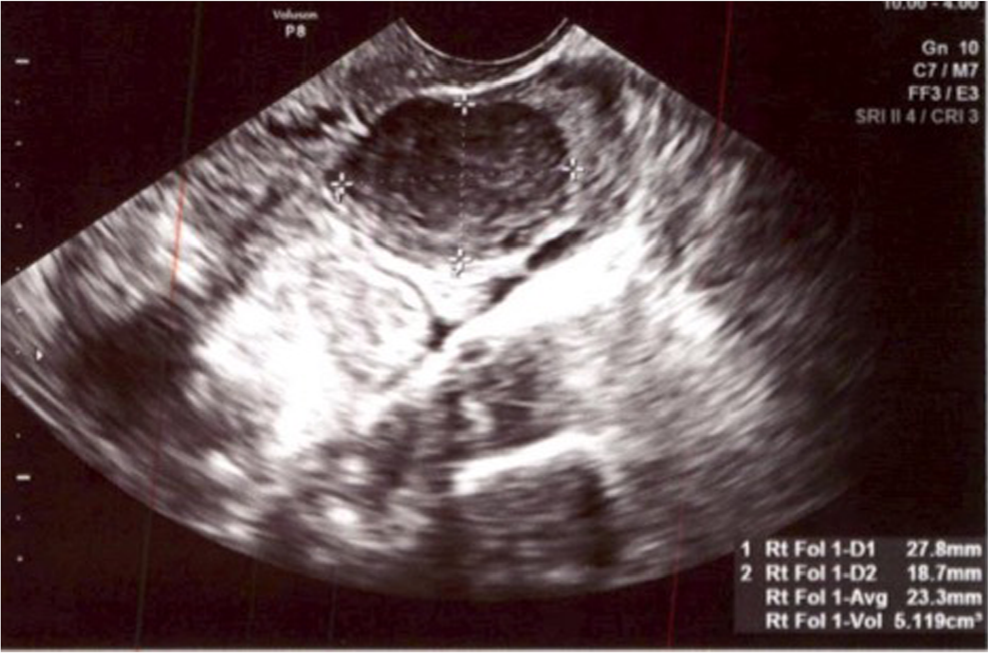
Case 1. Right ovarian mass presumed to be an endometrioma during ultrasound at the patient’s initial presentation. This homogeneous relatively uniform ovoid mass was initially thought to represent an ovarian endometrioma, instead of the granulosa cell tumor it proved to be
